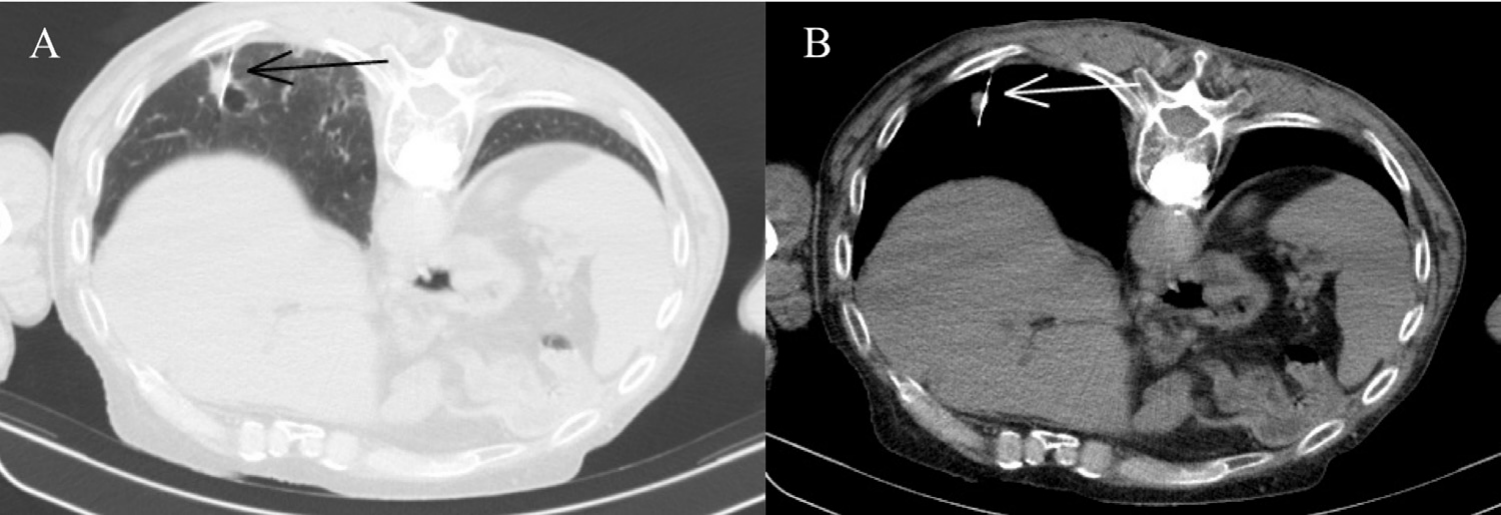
CT showing the percutaneous posterior intercostal access with the patient in prone position. Black (A) and white (B) arrows indicate the needle in the target lesion.
